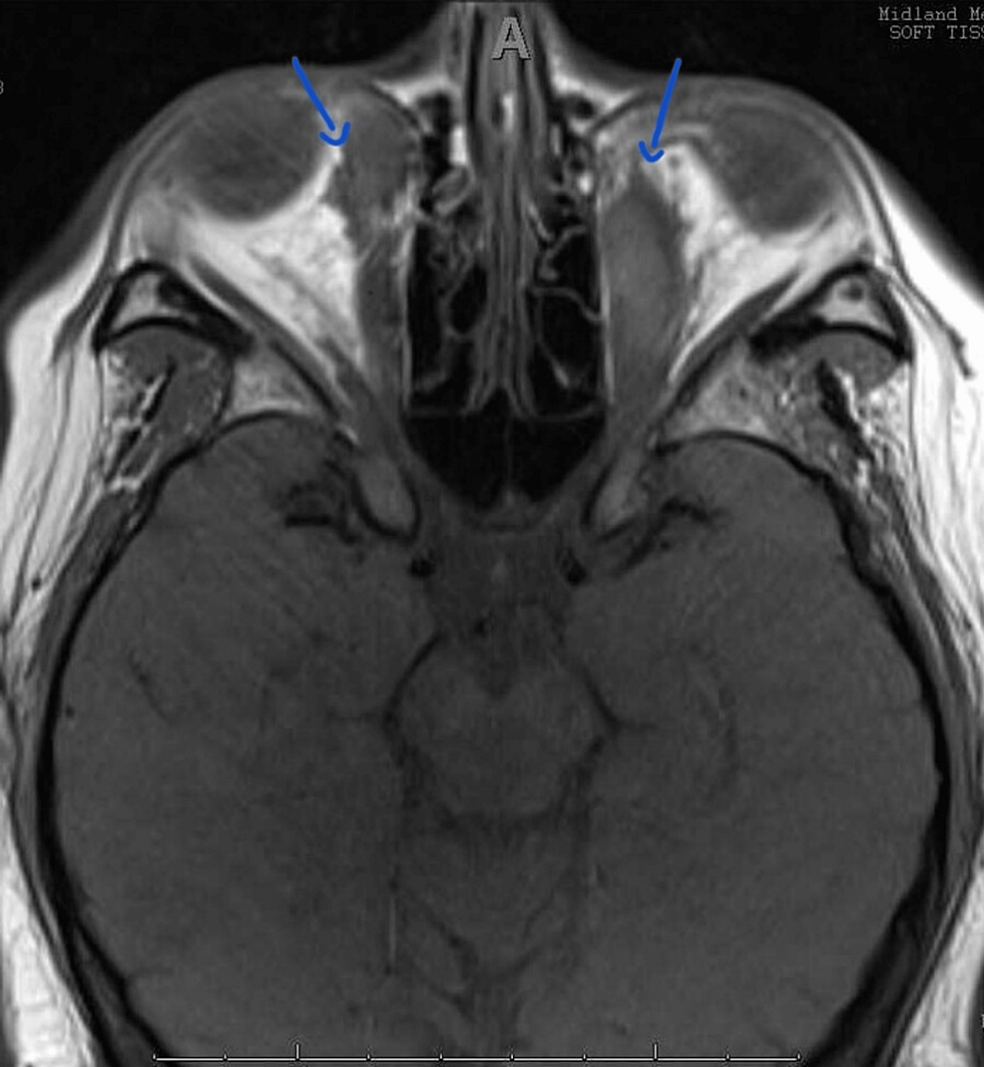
Post-treatment orbital MRI (axial view) showing significant reduction in enlargement of extraocular muscles (bilateral medial recti indicated by blue arrows) MRI: magnetic resonance imaging
